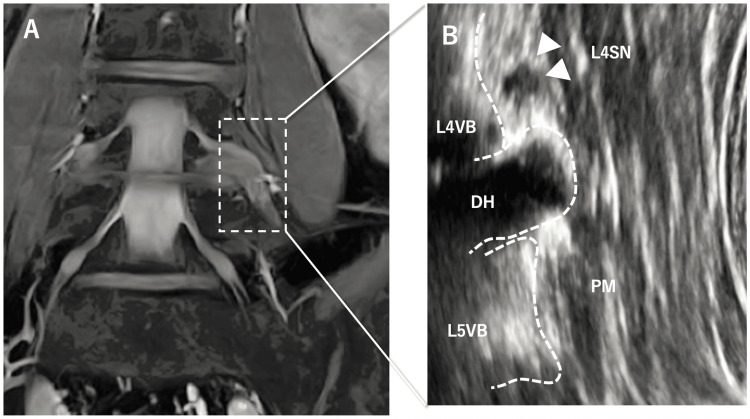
Magnetic resonance imaging (MRI) of the herniated disc and long-axis ultrasonographic images. A: Coronal MRI of the lumbar spine. B: Long-axis ultrasonographic image showing the L4 spinal nerve cranial to the herniated disc. L4VB, L4 vertebral body; L5VB, L5 vertebral body; L4SN, L4 spinal nerve (white arrow); DH, disc herniation; PM, psoas major muscle.
